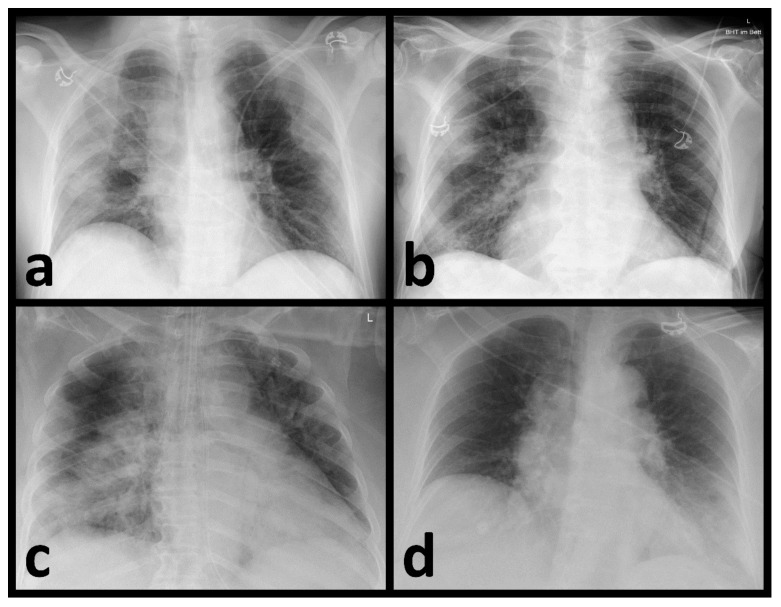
Chest radiographs with the distinguished distribution patterns regarding the probability of COVID-19 infection. (a) Typical (bilateral, peripheral opacifications), (b) almost typical (unilateral, peripheral opacifications), (c) non-typical (limited to one pulmonary lobe consistent with a lobar pneumonia) and (d) indeterminate (opacifications that could not be clearly classified as typical, almost typical, or non-typical).
